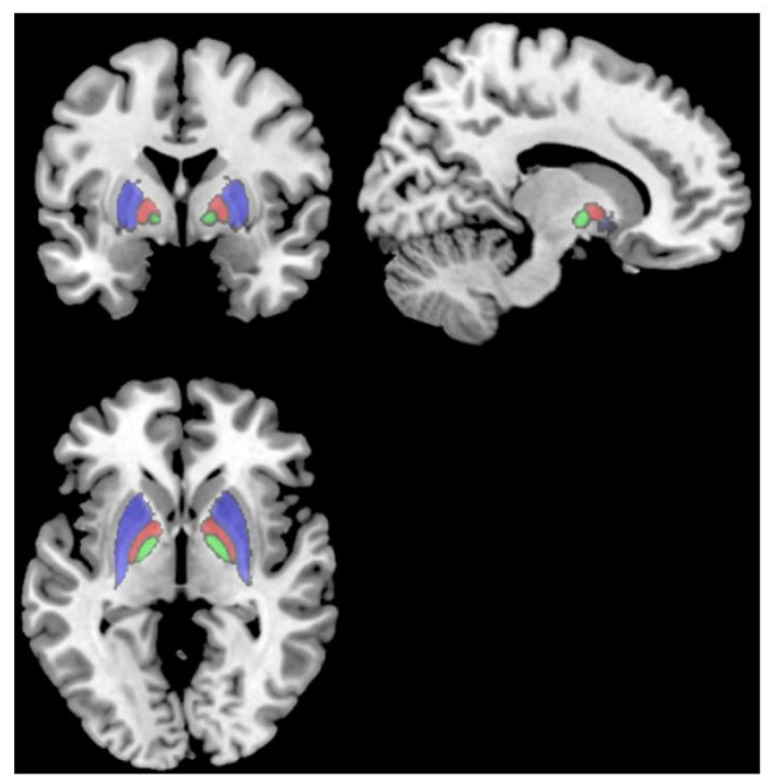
T1 MRI showing the putamen (red), pallidum (cyan), and thalamus (green), which all had smaller volumes in subjects > 6 months post-infection than subjects < 6 months post-infection [23].
